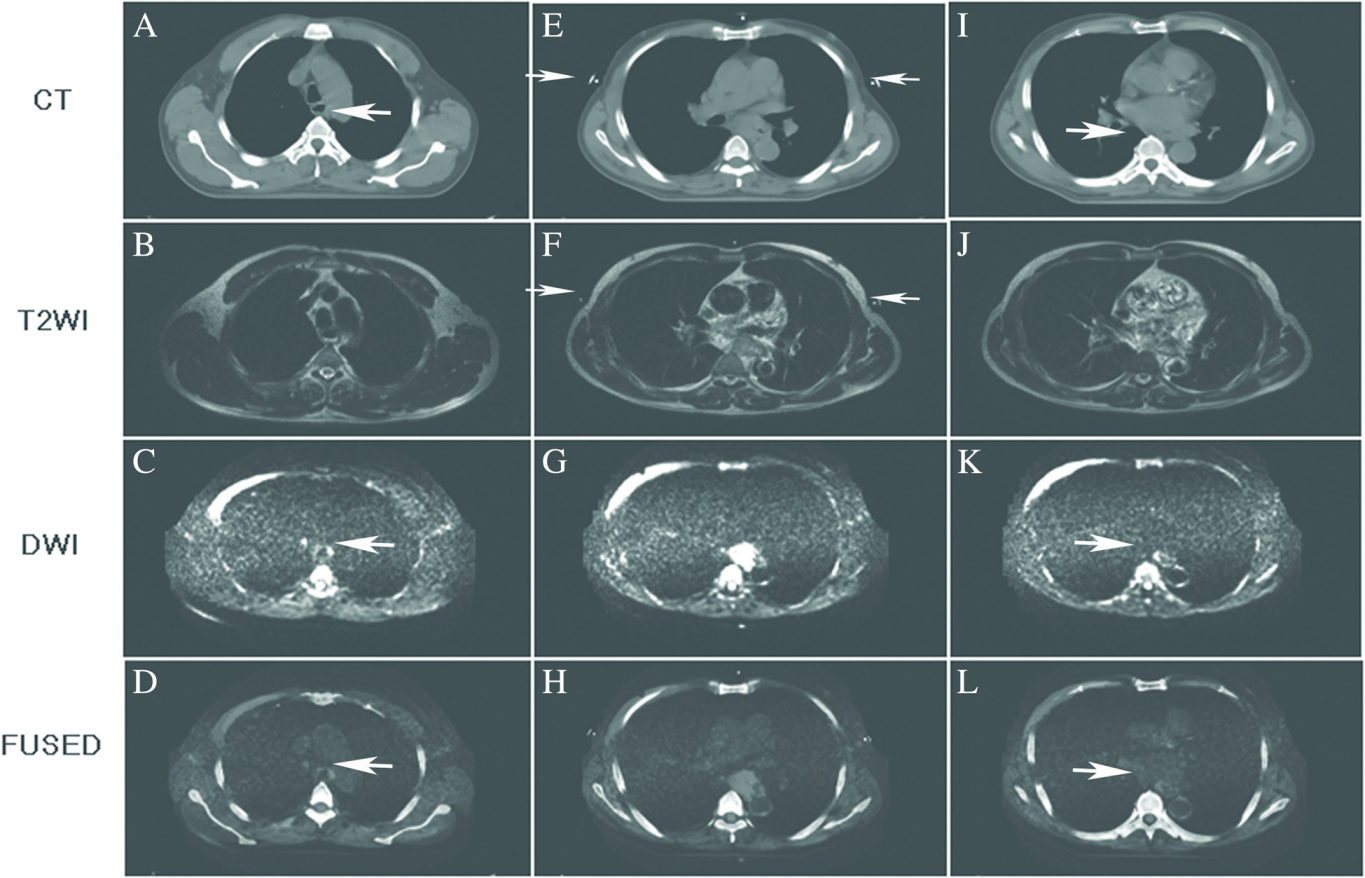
Different images show the GTV upper boundary, the largest GTV slice and the GTV lower boundary. (A-D) GTV upper boundary (white arrow) on CT images, T2W images, DWI scans and the fused images; In C and D, the white arrow indicates the upper boundary on DWI scans and fused images. (E-H) largest GTV slice on images; In E and F, the white arrow indicates the modules on CT image and T2WI image. (I-L) GTV lower boundary (white arrow) on CT images, T2W images, DWI scans and fused images; In K and L, the white arrow indicates the lower boundary on DWI scans and fused images.
